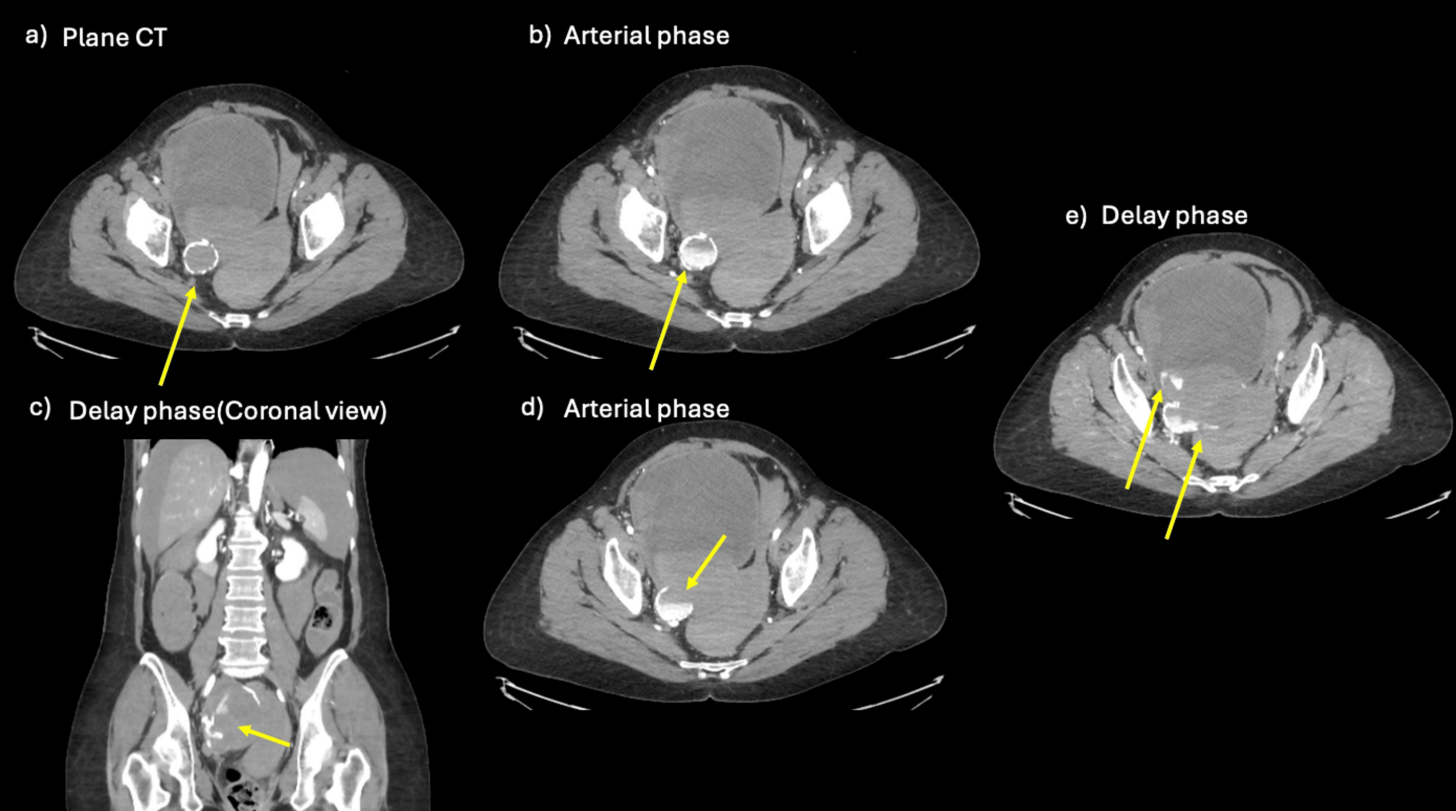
CT images on arrival at our hospital (a) A well-defined mass lesion with calcification is seen (arrow). (b) CT in the arterial phase shows contrast enhancement within a mass lesion containing calcification (arrow). (c) Contrast-enhanced CT (delayed phase) shows that the extravascular leakage has enlarged (arrow). Intra-abdominal hemorrhage is also evident. (d) Contrast-enhanced CT (arterial phase) shows that one part of the lesion lacks calcification, and extravascular leakage is seen external to the calcified area (arrow). This is a finding suggestive of a ruptured pseudoaneurysm. (e) Contrast-enhanced CT (delayed phase) shows that the extravascular leakage has further enlarged (arrow). The extravascular leakage is more evident than in Figure 1, and a diagnosis of rupture of a pseudoaneurysm of the right uterine artery was made.
